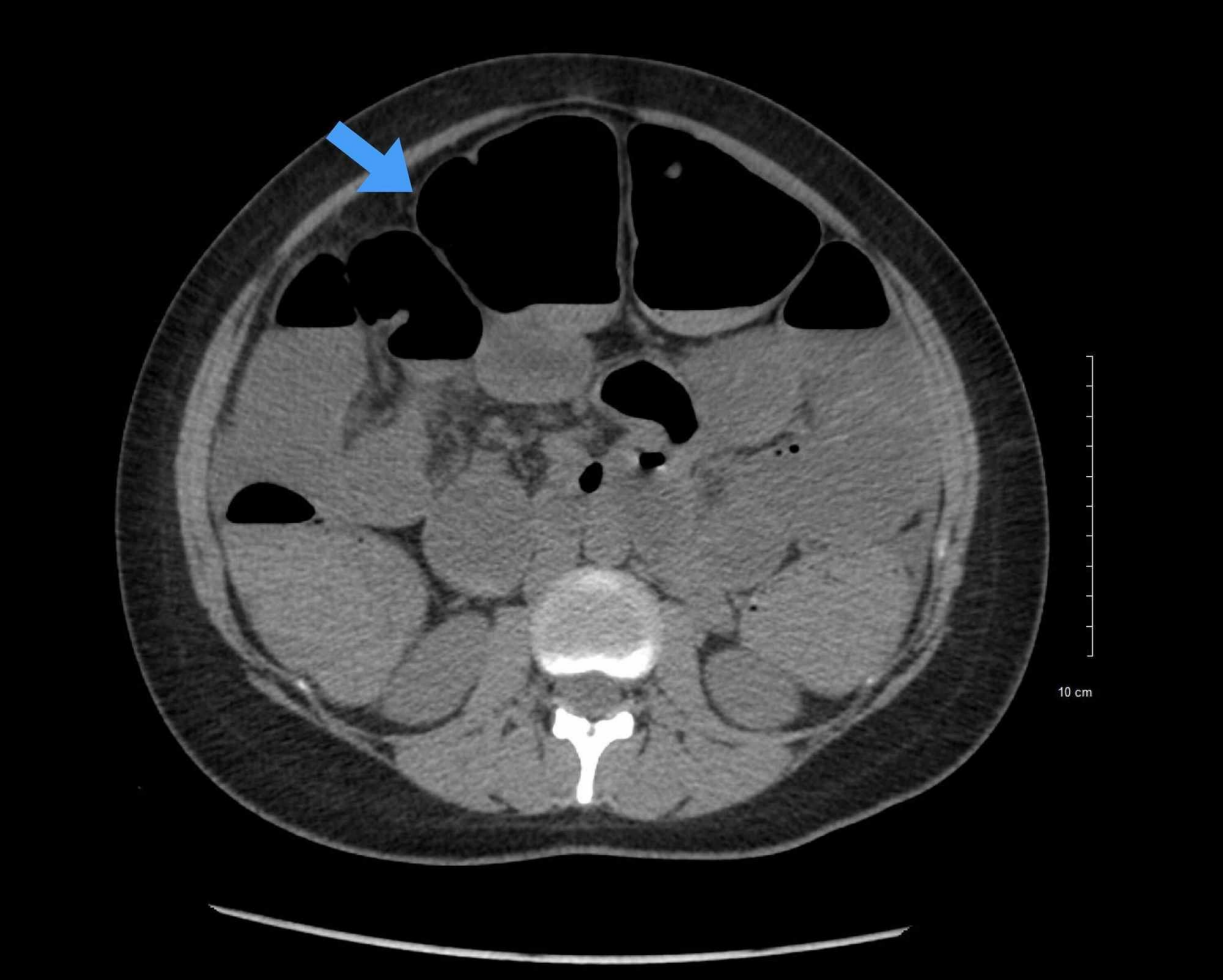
Axial slice from CT scan of the abdomen demonstrating dilated small bowel loops with air-fluid levels
